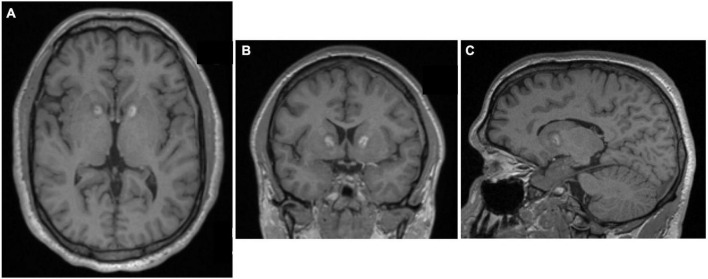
Laser interstitial thermal therapy (LITT) capsulotomy for OCD. (A) Axial, (B) Coronal, and (C) Sagittal MPRAGE MRI images after MR-guided LITT. Original figures kindly provided by Dr. Wael F. Asaad, Brown University.
